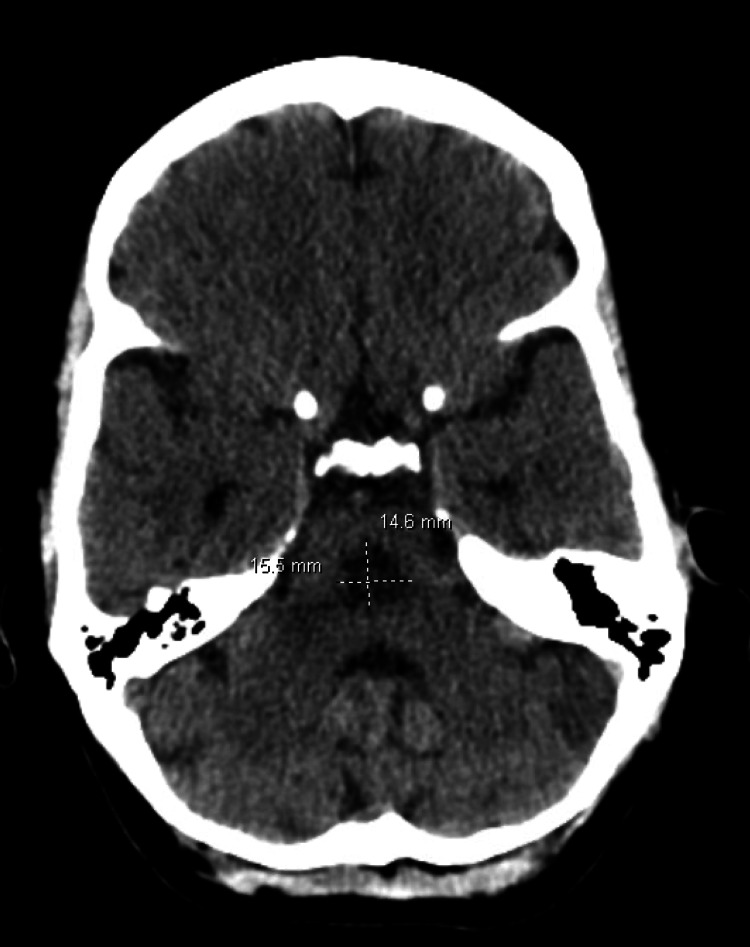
Computed tomography (CT) of the head
